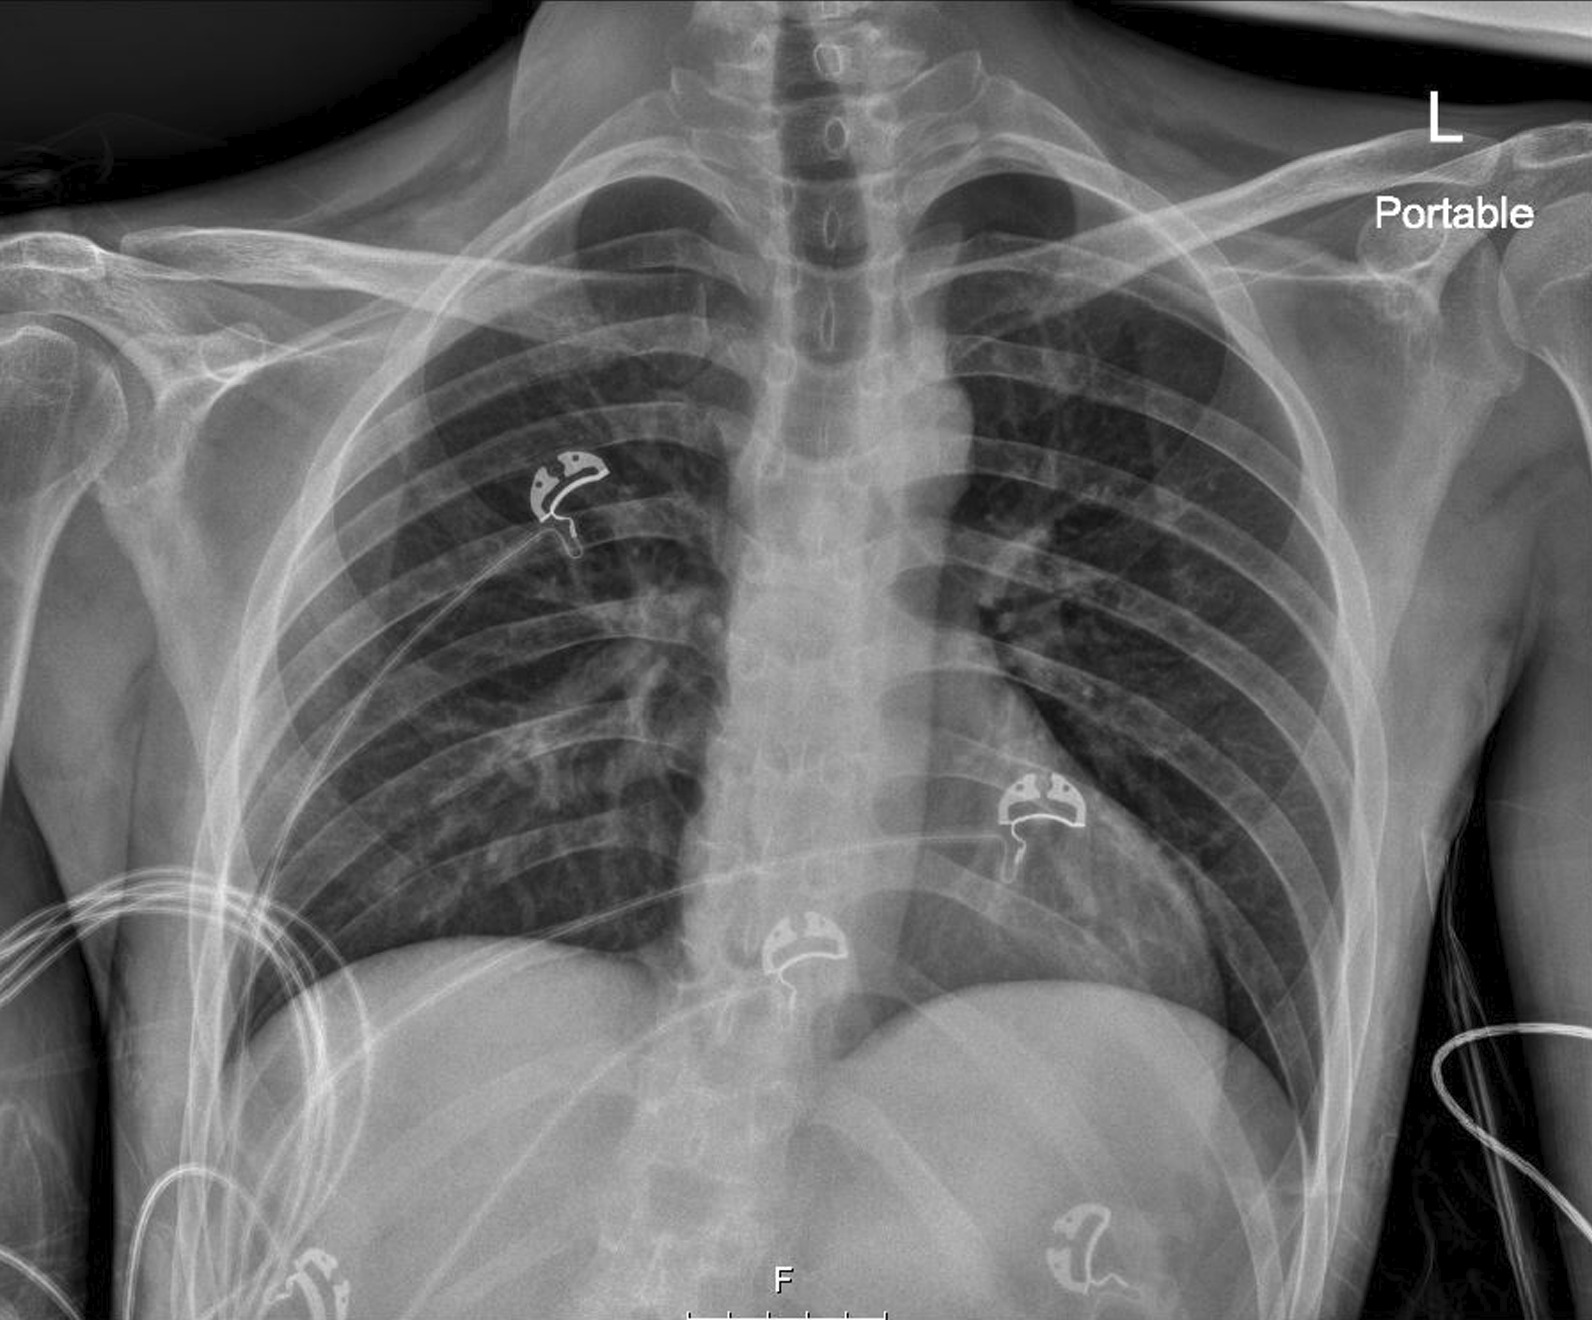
Chest radiograph showing absence of serious complication after prompt removal
